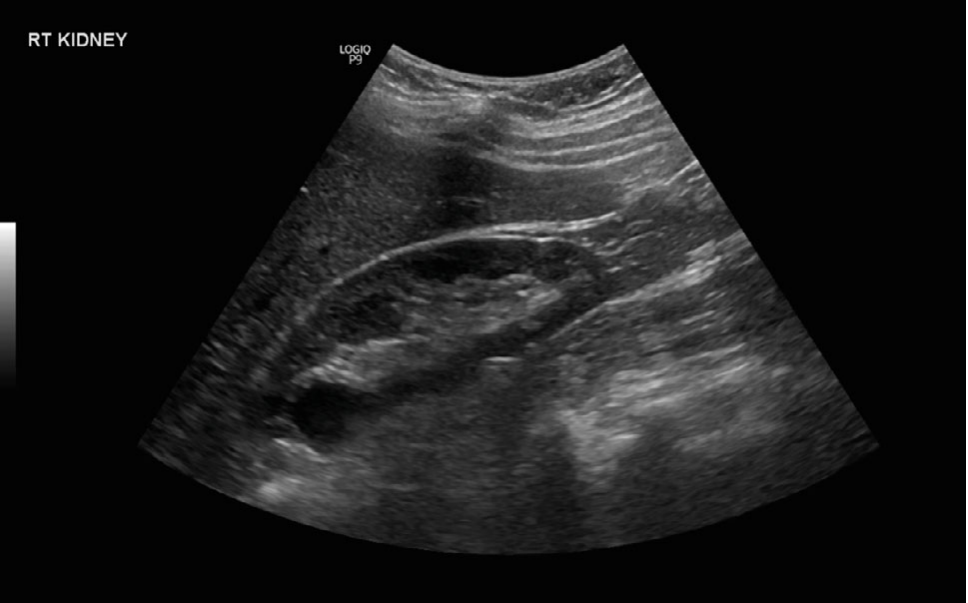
Right kidney ultrasound demonstrating normal size and echogenicity with preserved corticomedullary differentiation.
